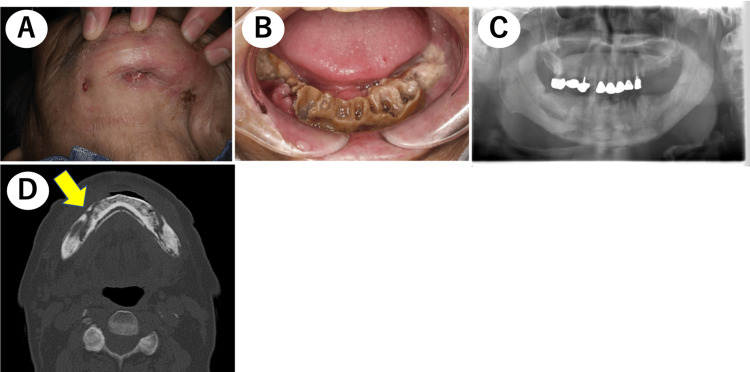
Clinical and radiographic findings before the treatment. (A) The extraoral examination revealed a fistula on the skin of the right mandibular area.
